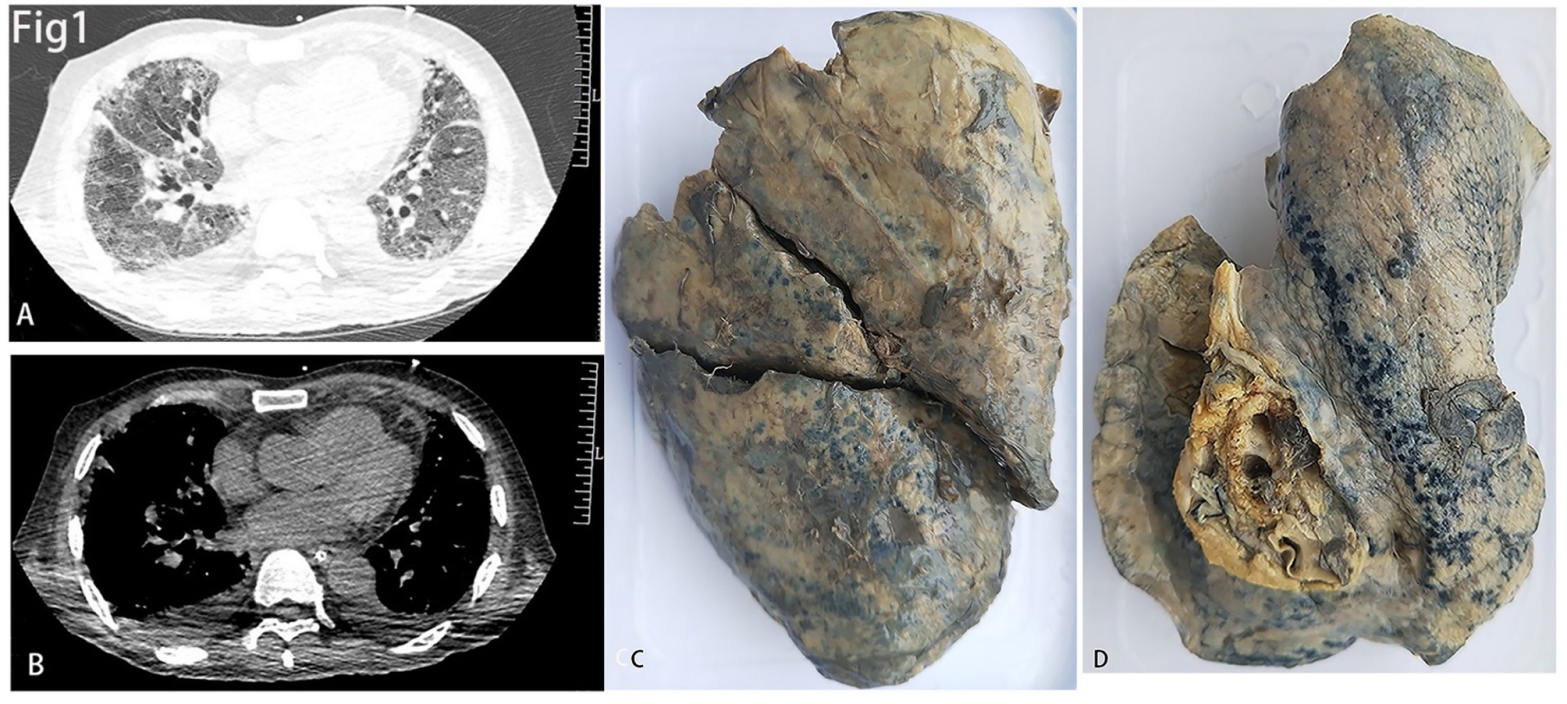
(A, B) The lung window (A) and mediastinal window (B) of CT showed diffused fibrosis of both lungs. (C, D) showed decreased size of the right (C) and left (D) lungs.
